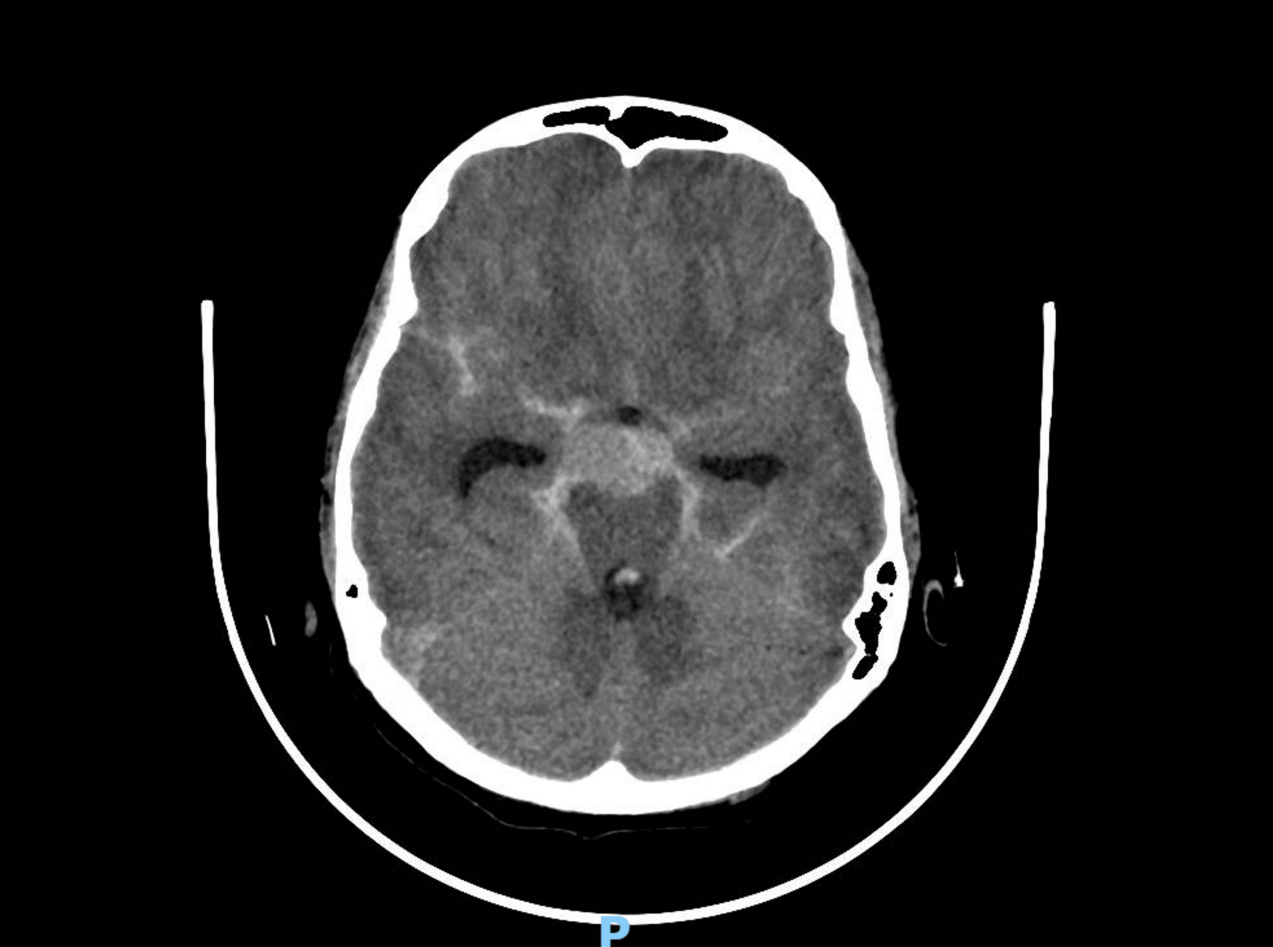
Non-contrast CT brain (axial view) demonstrating hyperdensity within the basal cisterns consistent with acute subarachnoid hemorrhage, with associated mild dilatation of the temporal horns
